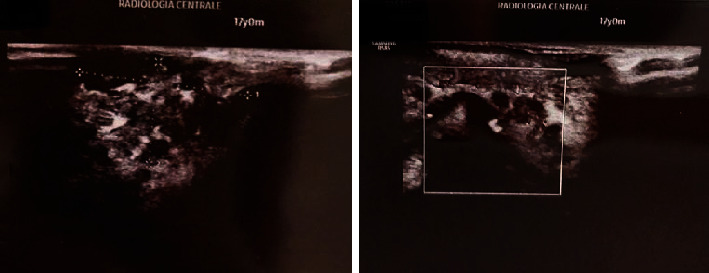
Color Doppler US imaging confirms the diagnosis of venous malformation.
